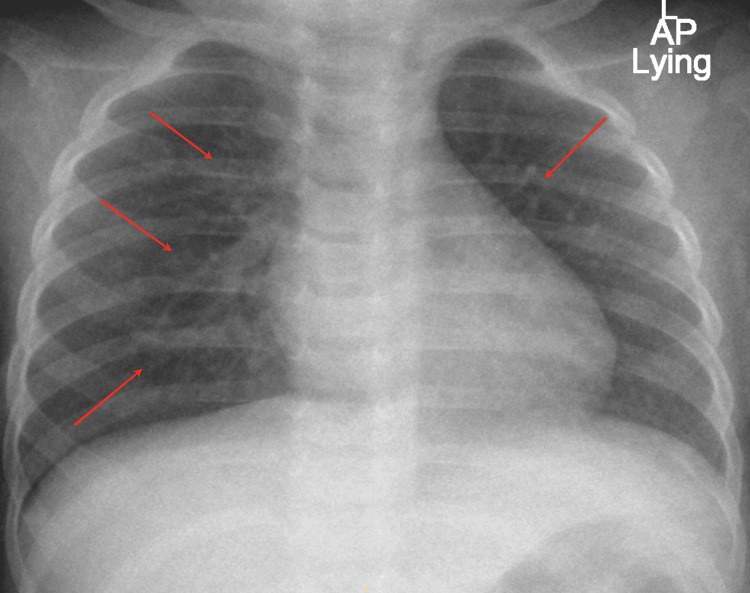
Chest X-ray showing prominent broncho-vascular markings suggesting a picture of bronchiolitis Red arrows pointing at prominent broncho-vascular markings
